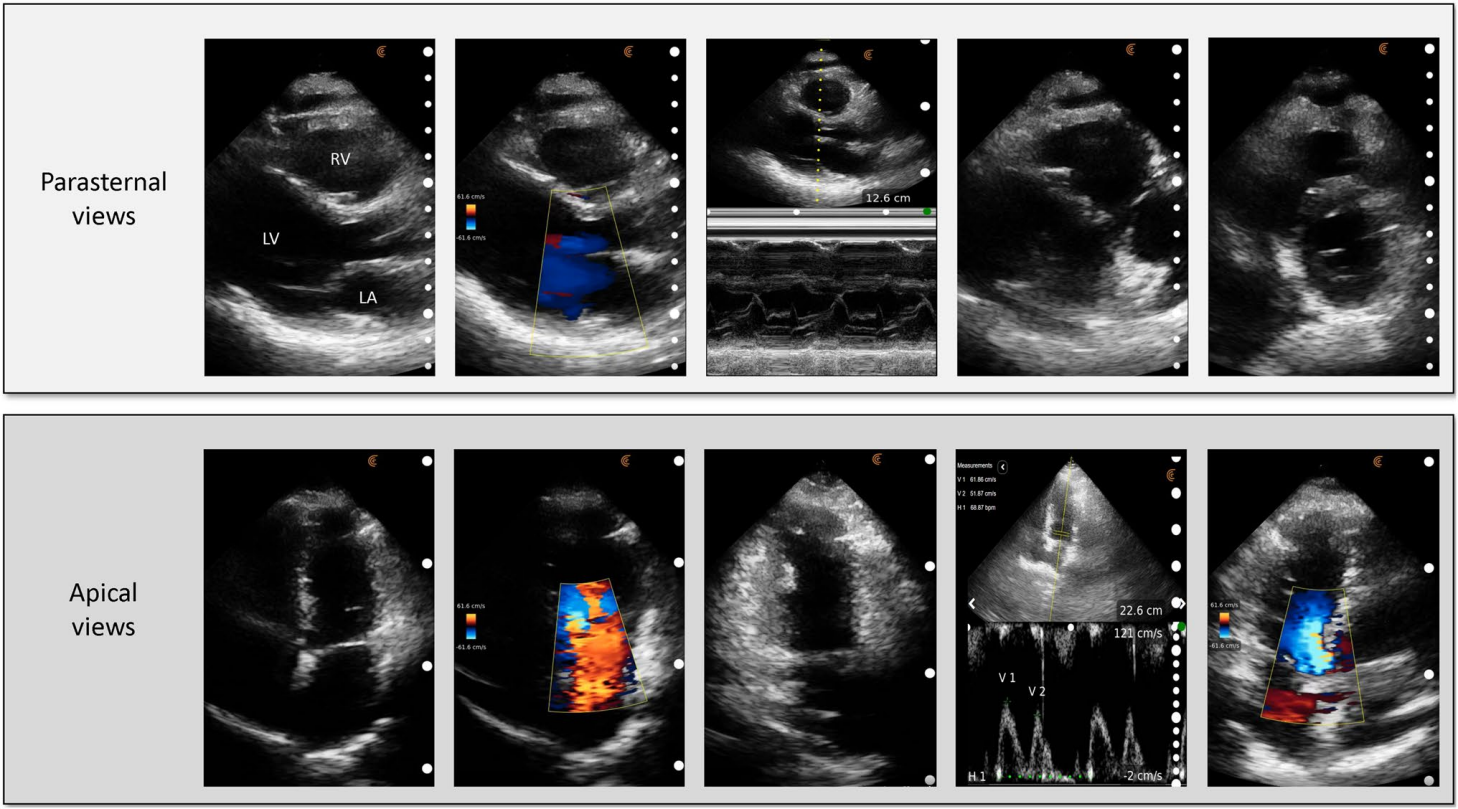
Comprehensive cardiac examination by Clarius PA HD. B-mode, colour Doppler and M-mode are demonstrated in parasternal views. In apical views, similar views and pulse wave Doppler for mitral inflow are demonstrated. V1 = peak early filling velocity, V2 = peak late filling velocity
